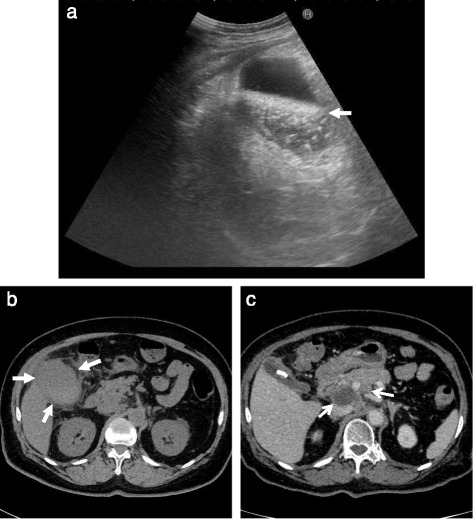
Ultrasonographic finding. a The gallbladder was filled with many small stones and sludge (*white solid arrow*). Computed tomographic findings. b Computed tomography (CT) showing a thick-walled and enlarged gallbladder (*white solid arrows*). c After percutaneous transhepatic gallbladder drainage, contrast-enhanced CT showing multiple round nodular lesions, which were suggestive of metastatic lymph nodes (*white arrows*)
